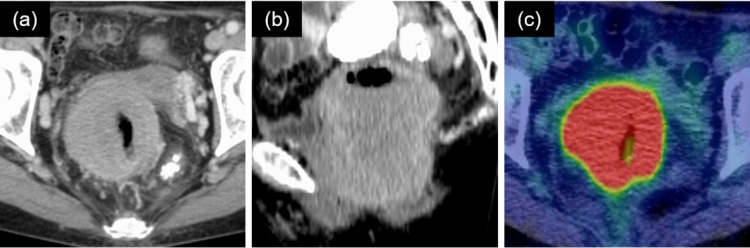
Images before radiotherapy. (a) Axial view of CT. (b) Sagittal view of CT. (c) Axial view of FDG-PET/CT. SUVmax: 20.5. CT: computed tomography; FDG-PET/CT: 18F-fluorodeoxyglucose-positron emission tomography/CT; SUVmax: maximum standardized uptake value
